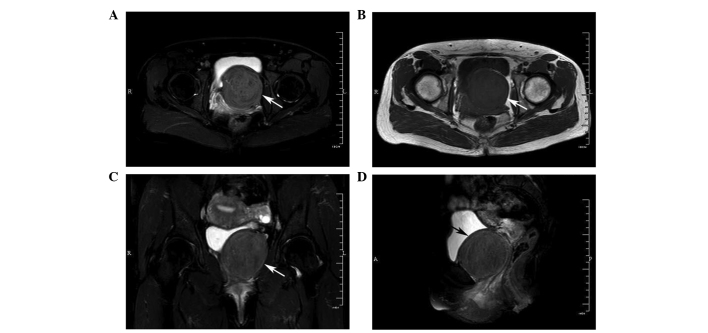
MRI showing the presence of the tumor on (A) T2-weighted and (B) T1-weighted images in the transverse plane. T2-weighted MRI showing (C) coronal and (D) sagittal planes. Arrows indicate the tumor. MRI, magnetic resonance imaging.
